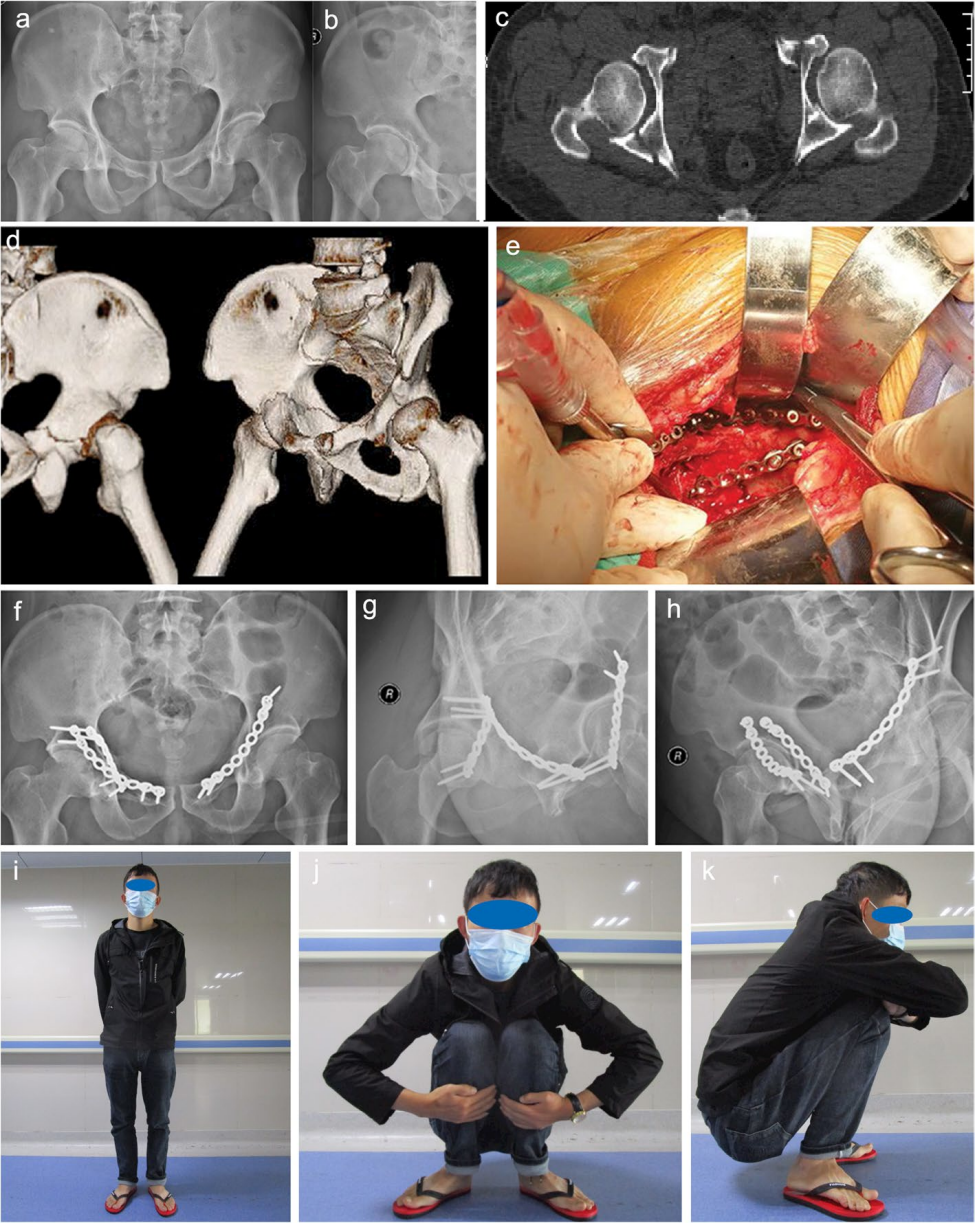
a, b X-rays of Case no. 2, who was involved in a fall from height resulting in a right T-shaped acetabular fracture combined with pelvic fracture. c The low level posterior column fracture line was evident in the plain and three-dimensional reconstruction computed tomography images. d Reduction and fixation were performed using the oblique-ilioischial plate through a single modified Stoppa approach. Postoperative radiographs of the anteroposterior view of the pelvis e, obturator oblique f, and iliac crest oblique g views showed a satisfactory reduction of the fracture m, n. The three-dimensional reconstruction shows the location of the implant o–q. Photographs at 9-months postoperatively show that satisfactory hip function was achieved
